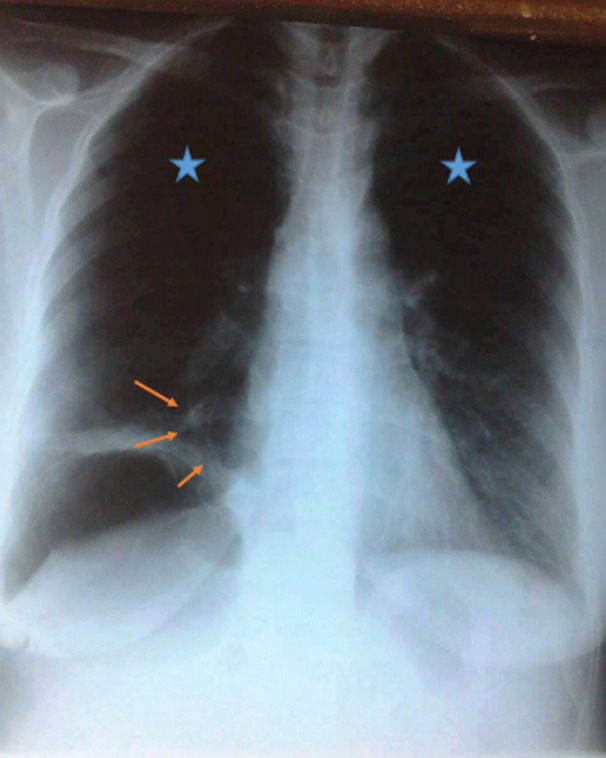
radiographie du thorax: atélectasie du lobe inférieur droit (au niveau de la flèche orange) avec emphysème obstructif (étoile bleue)
